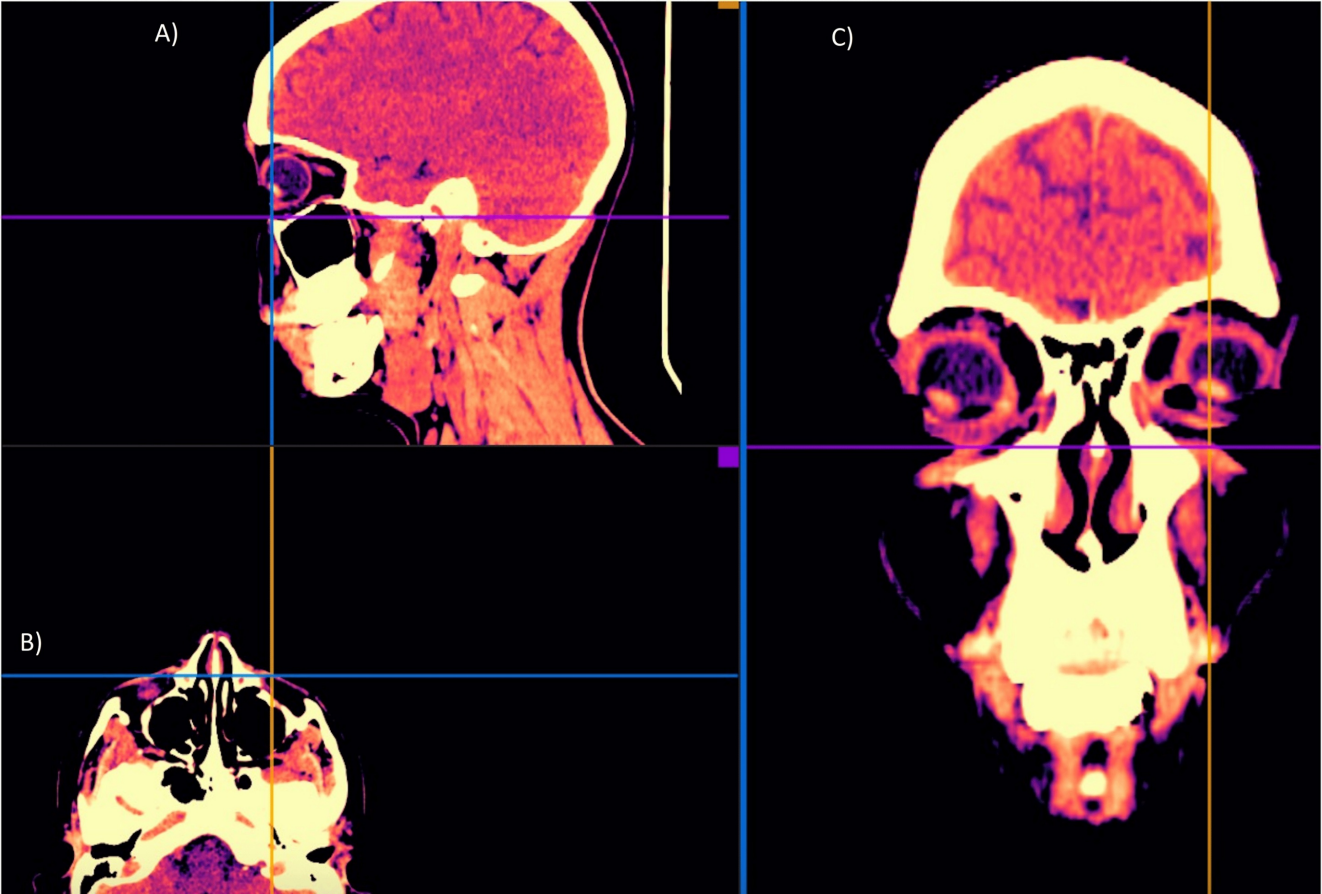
Facial CT multiplanar reconstruction (MPR) right lacrimal sulcus or multiplanar reconstruction. In these images it was possible to demonstrate in the three planes of space through a sagittal (A), coronal (B) and axial (C) views that Juvelook did not alter the tissue density or radiological CT pattern of the tissues after having been applied (two weeks post injection).
